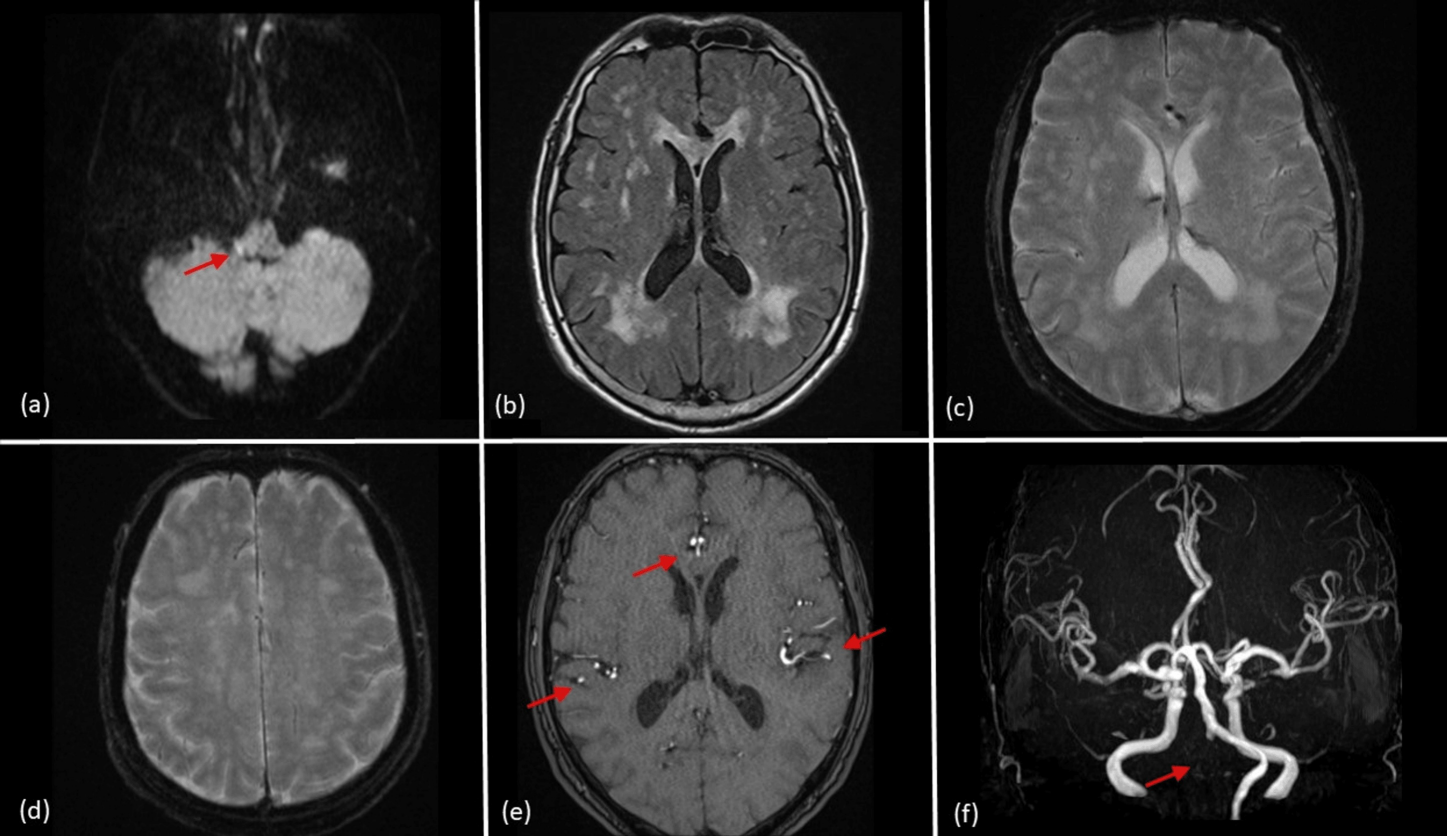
Initial MRI findings on hospital admission. (a) Diffusion-weighted imaging demonstrates acute ischemic changes in the right brainstem, with restricted diffusion in the right vertebral artery territory (arrow). (b) Fluid-attenuated inversion recovery image shows chronic ischemic changes in cerebral white matter. (c) T2-weighted imaging (T2*-WI) depicts no abnormal venous or arterial engorgement at this stage. (d) Another slice of T2*-WI shows no significant vascular abnormalities. (e) The source images of magnetic resonance angiography (MRA) show normal arterial blood flow signals except for the right vertebral artery (arrows). (f) Maximum intensity projection of the MRA highlights the absence of flow in the right vertebral artery, correlating with brainstem ischemia (arrow).
